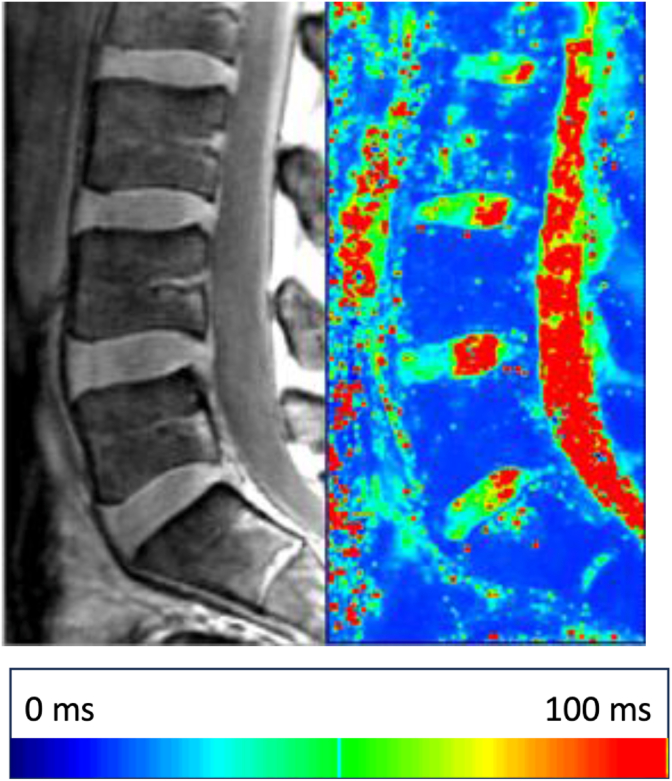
(Left) Midsagittal reformat of T2∗-weighted morphological magnetic resonance imaging and (right) the corresponding midsagittal T2∗ map. Note: High T2∗ values can be observed among L2/3 - L5/S1 as signs of healthy IVDs.
